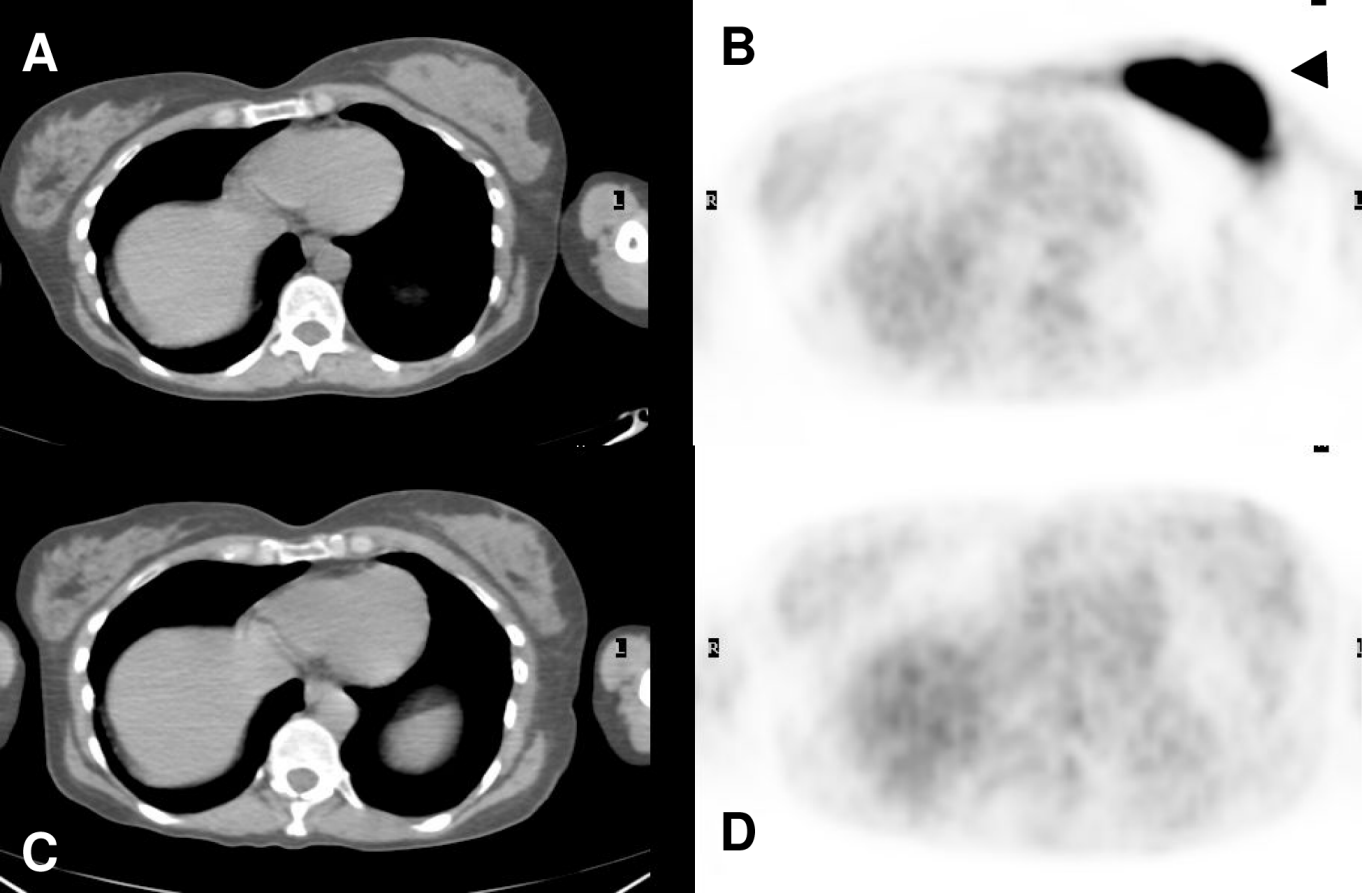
(A) Axial computed tomography (CT) and (B) axial positron emission tomography (PET) images of pre-treatment PET/CT scan showed an intense FDG avid mass in the left breast (arrowhead). (C) Axial computed tomography (CT) and (D) axial positron emission tomography (PET) images of post-treatment PET/CT, three months after completion of chemotherapy, showed resolution of previously fludeoxyglucose (FDG) avid breast lesion.
